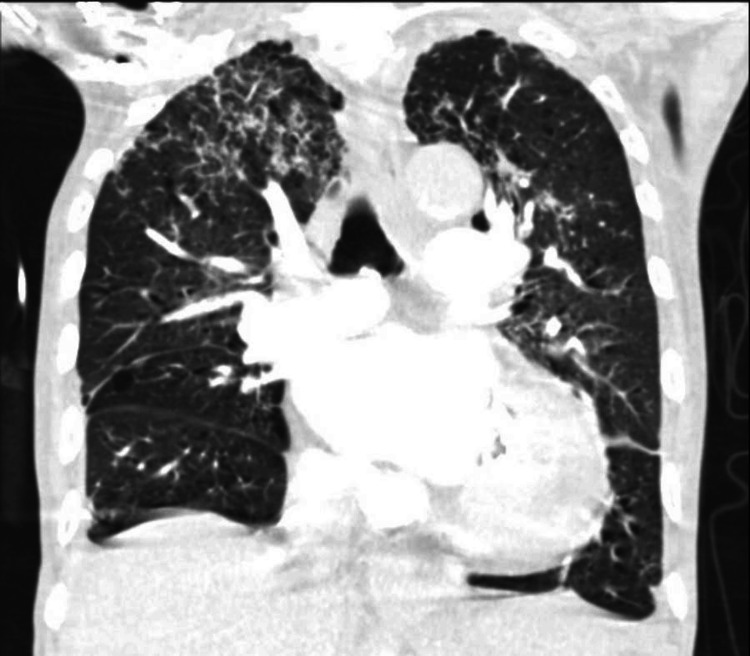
Coronal CT chest with upper-lobe predominant abnormalities
